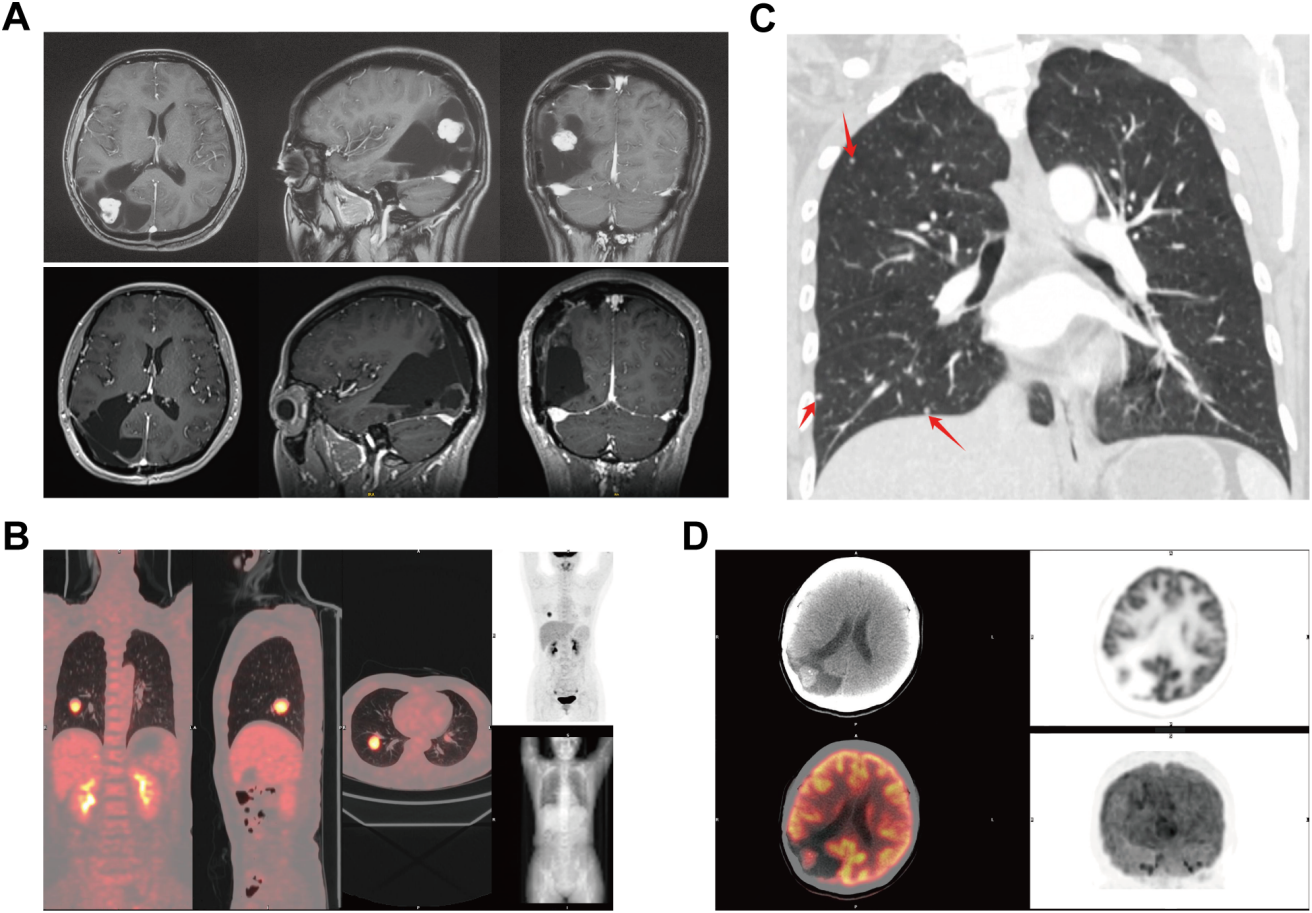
Imaging of recurrent meningioma in the head and body. (A) Pre - and post-operative enhanced MRI of the head. (B) The largest density nodule shows localized increased metabolism. (C) Multiple nodular shadows in both lungs (indicated by red arrows) distributed in the peripheral lung zones. (D) PET-CT showing increased metabolic activity in the right parieto-occipital lesion.
